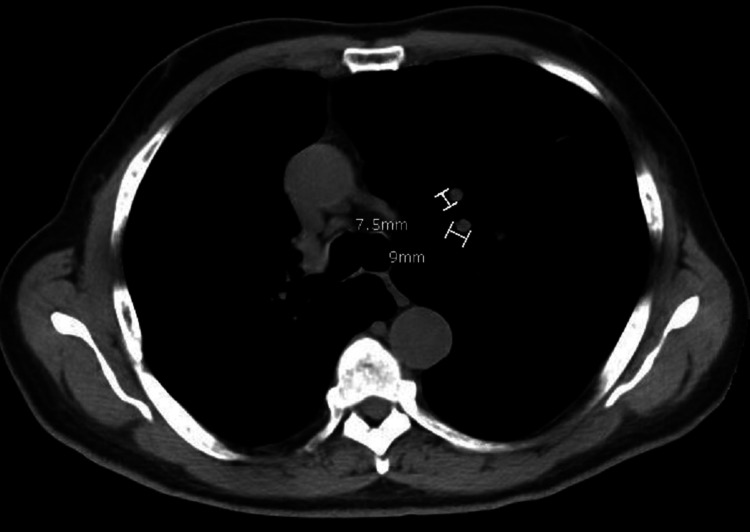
Chest CT scan demonstrating two new left lung nodules
